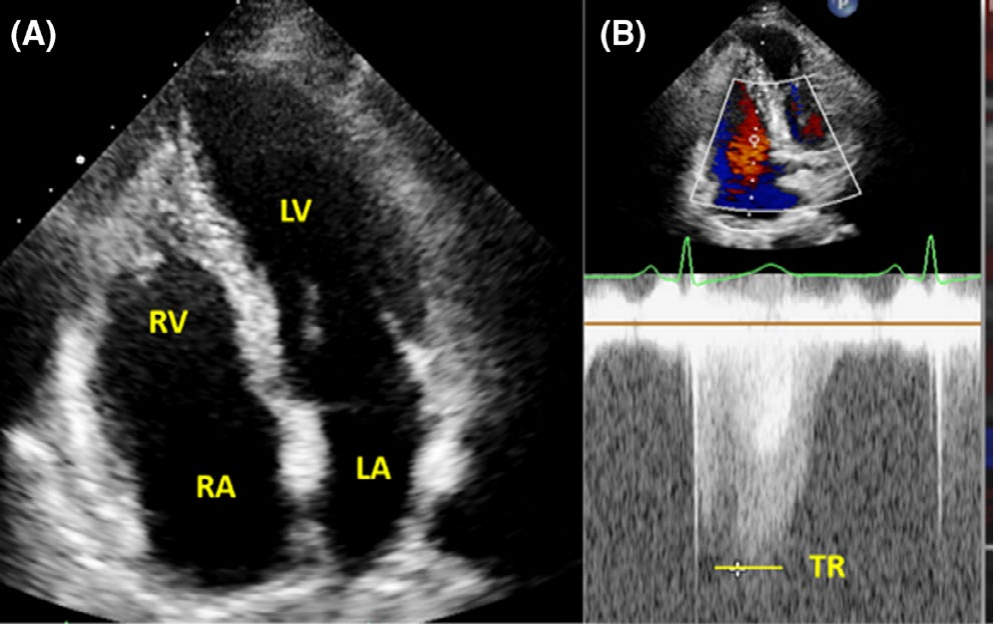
In this echocardiographic image, (A) right ventricular 4‐chamber view shows a mildly enlarged right ventricle and (B) the continuous wave Doppler of the tricuspid jet indicates a mildly increased tricuspid regurgitation velocity
